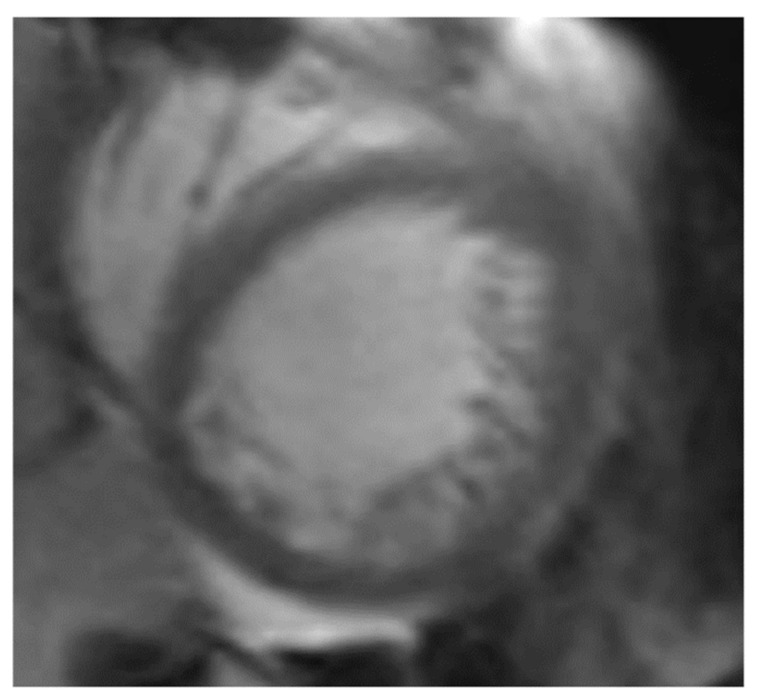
Magnetic resonance imaging: short axis of the left ventricle with excessive trabeculations. Notable, the septum shows no trabeculation.
